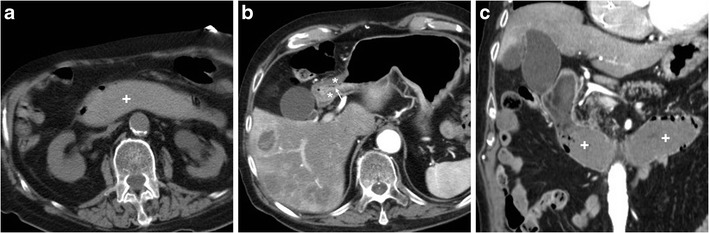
In an elderly 84-year-old male with haematemesis and melaena, precontrast CT scans (a) showed dilated second, third and fourth duodenum filled by hyper attenuating fluid (+) consistent with recent haemorrhage. Arterial-phase CT acquisition (b, c) showed characteristic appearance of antropyloric PUD with submucosal oedema (*) and mucosal hyperenhancement (thin arrow) but did not reveal signs of active bleeding, as confirmed by endoscopy
